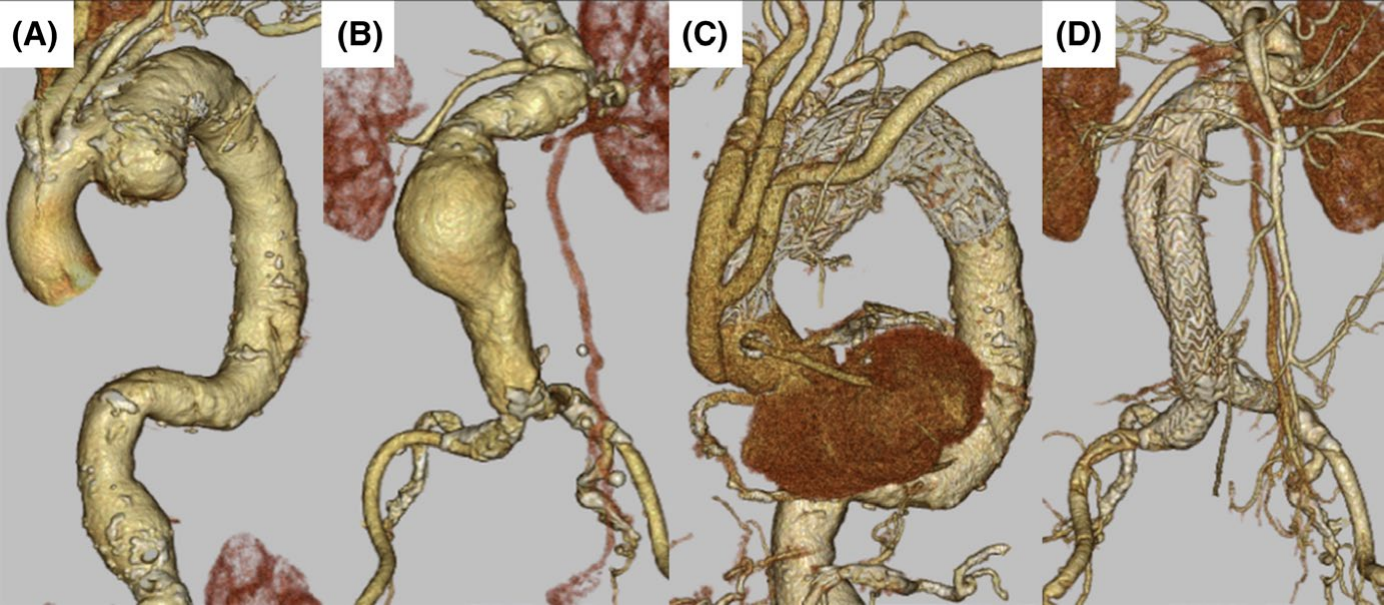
A CT angiogram reveals a saccular TAA (70 mm) (A) and an infrarenal AAA (65 mm) (B). The postoperative CT angiogram shows total debranching thoracic endovascular aortic repair of the TAA (C) and endovascular aortic repair of the AAA (D), excluding the aortic aneurysm. The patency of the great SVG is also confirmed (C). AAA, abdominal aortic aneurysm; CT, computed tomography; SVG, saphenous vein graft; TAA, thoracic aortic aneurysm
